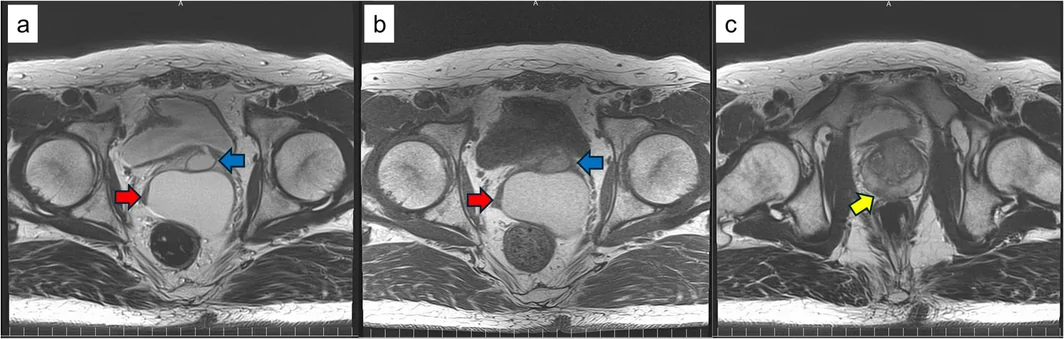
Red thick arrow indicates the suspected seminal vesicle cyst. Blue thick arrow, structure suspected to represent the ejaculatory duct. (a) MRI T2‐weighted image. The mass suspected to be a seminal vesicle cyst measured approximately 6 cm in diameter and demonstrated high signal intensity. It was continuous with a structure suspected to represent the ejaculatory duct. (b) MRI T1‐weighted image. The mass suspected to be a seminal vesicle cyst demonstrated relatively high signal intensity, suggesting that the internal fluid was viscous, such as mucin, or hemorrhagic. (c) MRI T2‐weighted image. A low‐signal‐intensity lesion was identified in the peripheral zone of the right lobe of the prostate, raising suspicion of malignancy (yellow thick arrow).
